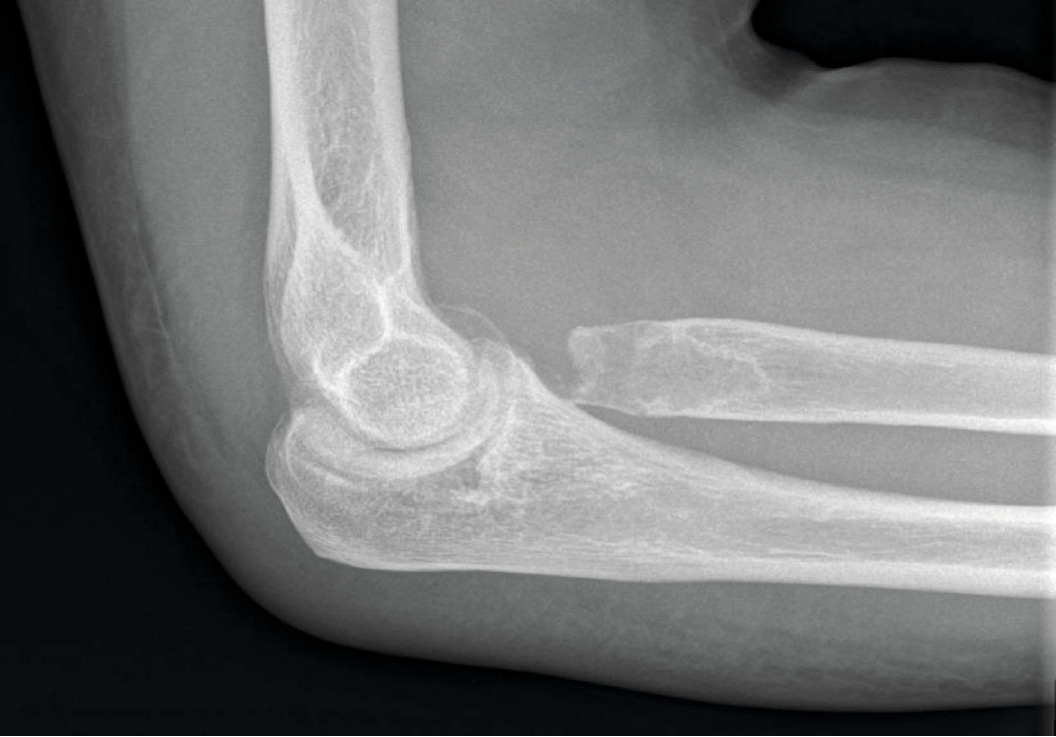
Lateral X-ray of the elbow after removal of the silastic radial head prosthesis.
